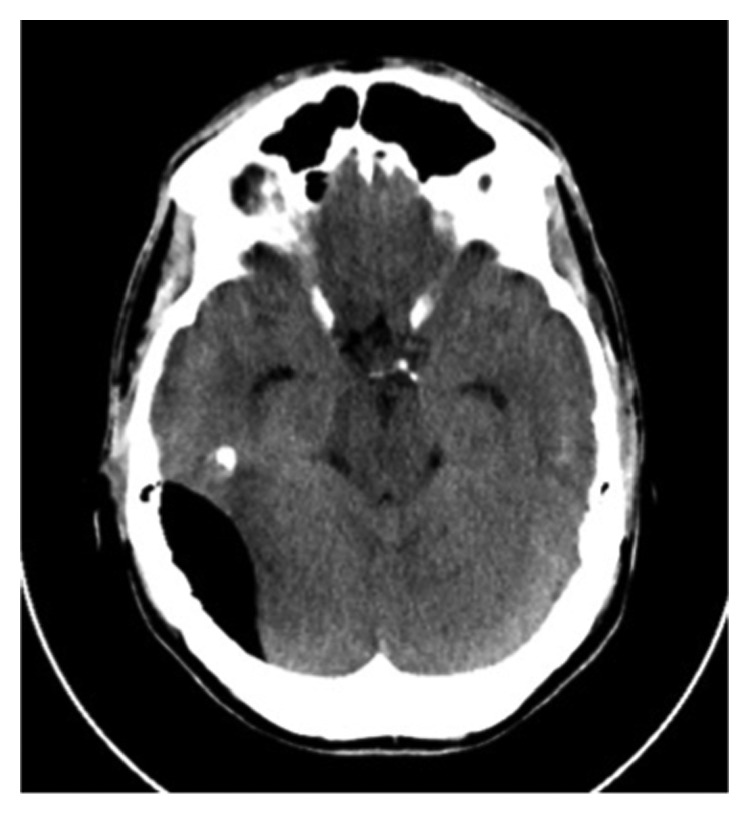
CT head demonstrating right-sided pneumocephalus.
